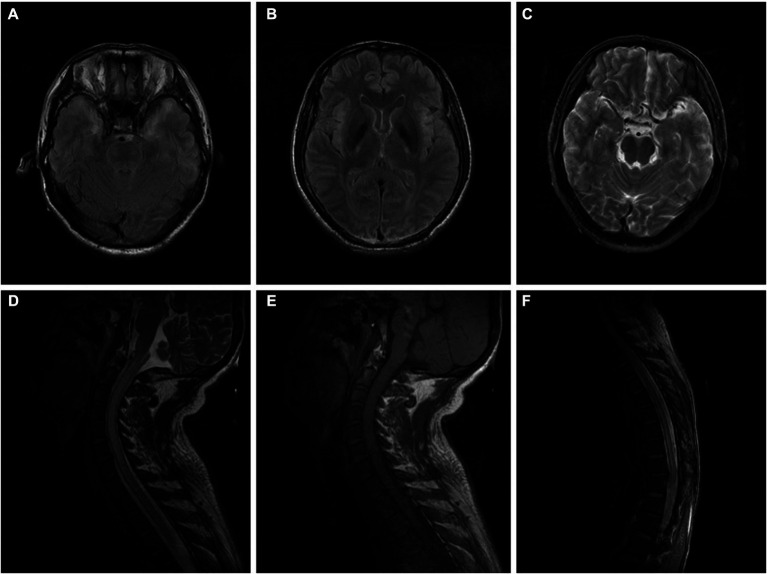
(A–C) Brain MRI of the patient in case 1 indicated high signal intensity of bilateral temporal lobes and insular cortex in a T2WI sequence performed without enhancement on a contrast-enhanced scan (10 days after onset). (D–F) Cervicothoracic MRI of the patient in case 1 showed extensive thickening of the cervical and thoracic spinal cord, especially the cervical spinal cord, and an increased signal intensity in T2WI sequence, with slightly patchy enhancement of the lesions on contrast-enhanced scan (10 days after onset).
